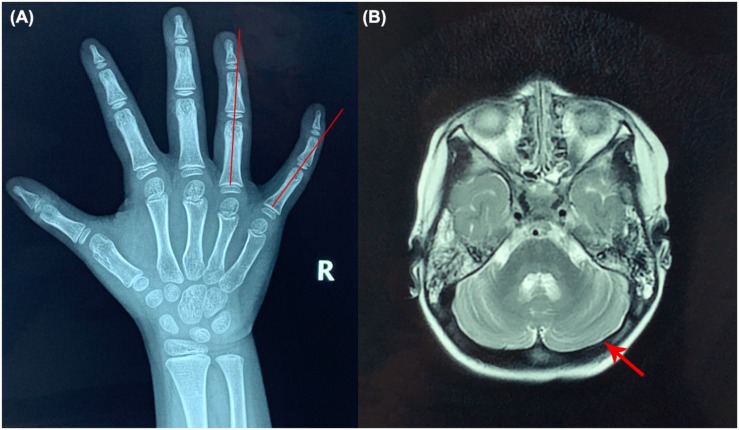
X-ray of the right hand and brain MRI of the patient. (A) Ulnar deviation of the right-hand ring finger and little finger (red lines). (B) Brain MRI results (red arrow shows the cerebella).
